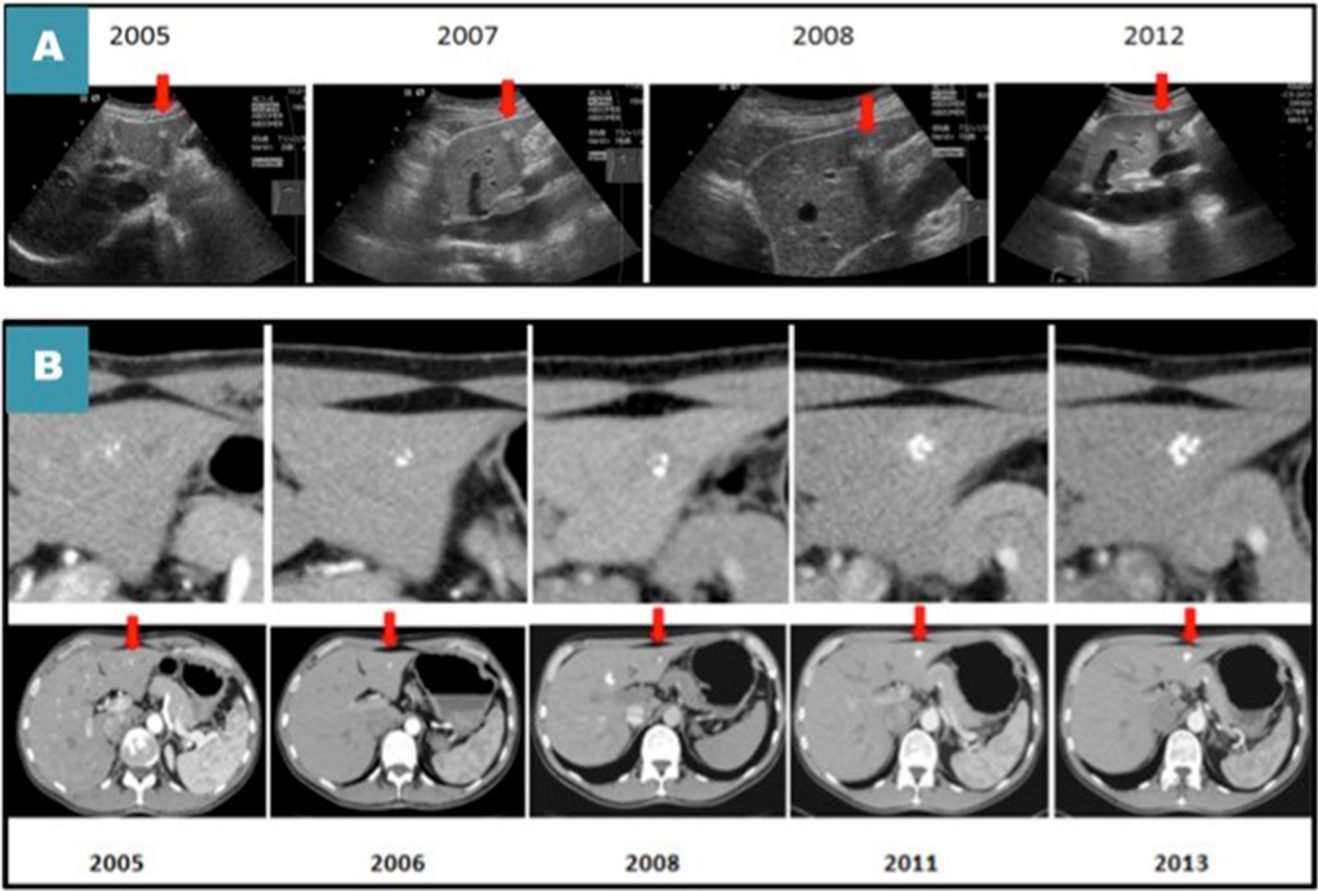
A metastatic lesion in segment 3 of the liver of a single patient in long term follow-up (CT-scan and ultrasound) demonstrating minimal growth over eight years (A) ultrasound (B) CT-scan with zoomed-in calcified lesion.
